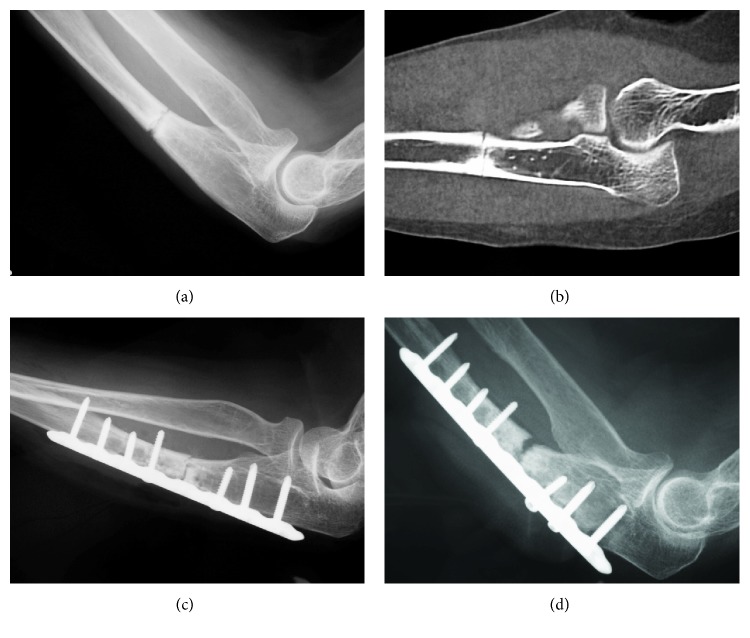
Imaging findings. Plain radiography (a) and computed tomography (b) images show transverse fracture at the proximal ulna. Plain radiographs immediately after open reduction with internal fixation (c) and at three months thereafter (d).
